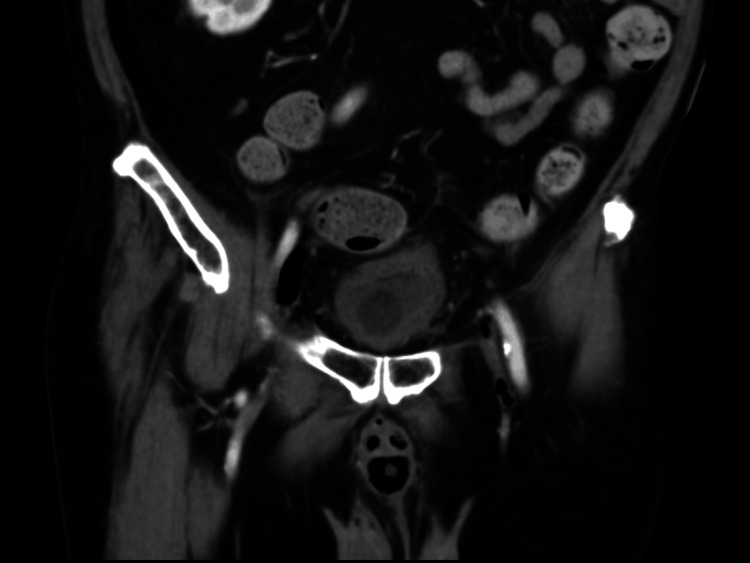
Contrast-enhanced CT coronal reconstruction image of the pelvis The image demonstrated bladder wall thickening, air in the corpus cavernosum/corpus spongiosum, air along the fascial planes of the pelvis, and small foci of air in the left common femoral vein.
